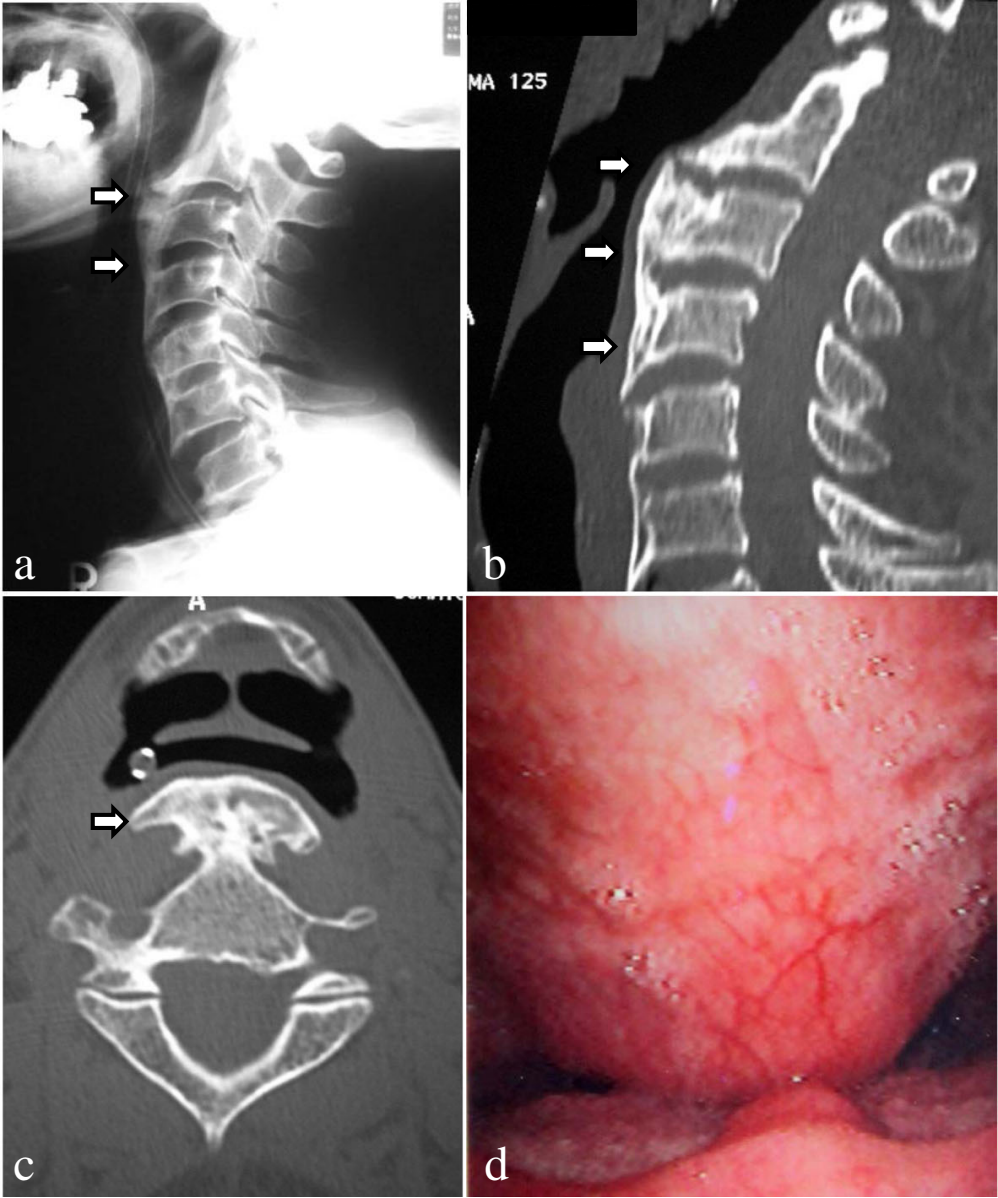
a A recurrent ossification of the anterior longitudinal ligament with anterior osteophytes (*arrows*) was present from C2 to C6 on a lateral radiograph taken 7 years after the first ossification of the anterior longitudinal ligament resection. b, c Sagittal and axial computed tomographic images of the cervical spine (C3, bone window) showed extensive ossification of the anterior longitudinal ligament with anterior osteophytes (*arrows*) displacing and deforming the contour of the proximal posterior pharyngeal wall. d Endoscopic examination revealed narrowing of the esophageal entrance by the ossification of the anterior longitudinal ligament with anterior osteophytes
